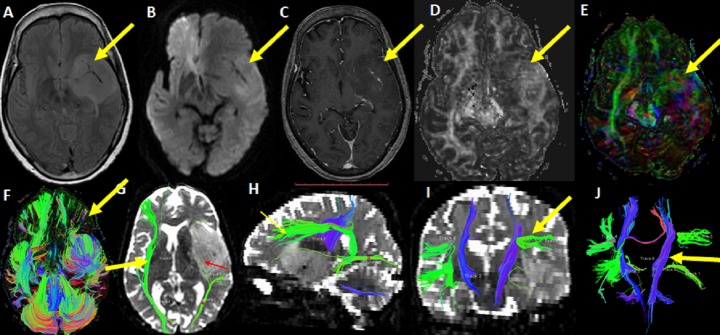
A 34-year-old female with left insular low grade glioma The FLAIR, (A) DWI (B), and post contrast T1-W (C) images show left insular lesion with no significant diffusion restriction as well as intralesional enhancement (arrows). Fractional anisotropy (FA) image (D) shows slightly- reduced FA. The colored map (E) shows distortion and destruction of white matter fibers in left frontal and temporal region (arrow). Axial whole brain tractography (F) shows destruction and distortion of the left frontal and insular fibers (arrow). 3-D tractography (G) shows destroyed left inferior frontal-occipital fasciculus (IFO) tract (red arrow) and intact right IFO (yellow arrow. 3-D tractography (H) shows anterior part of arcuate fasciculus (AF) in close relation to the anterior superior part of lesion (yellow arrow). (I) The leftcorticospinal tract (CST) is shown intact, away from lesion, yet medially deviated. 3-D tractography (J) of bilateral CST, AF, and IFO is shown.
